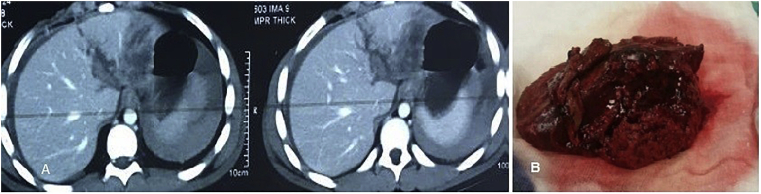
A) CT images of male patient, 12 years old, grade IV trauma to the left lateral sector of the left lobe, exploration after failure of conservative treatment revealed major bleeding from portal vein in the umbilical fissure and left lateral resection was done, then patient developed minor bile leak from the drain that resolved spontaneously. B) Operative image of the resected left lateral part.
